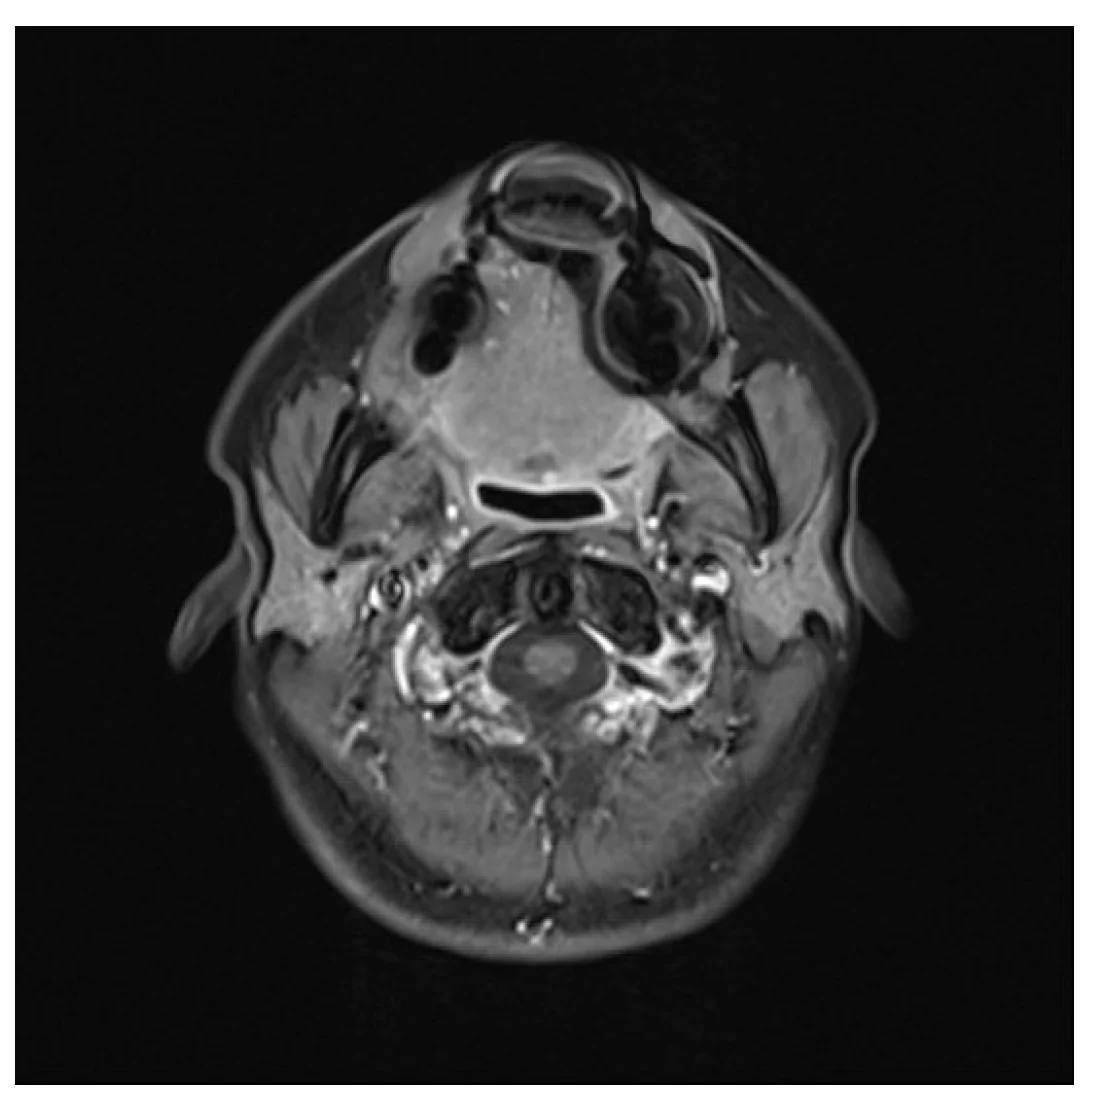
Follow-up magnetic resonance imaging (MRI) performed approximately 10 months after completion of radiotherapy, demonstrating regression of cervical lymphadenopathy with no obvious evidence of tumor recurrence.
